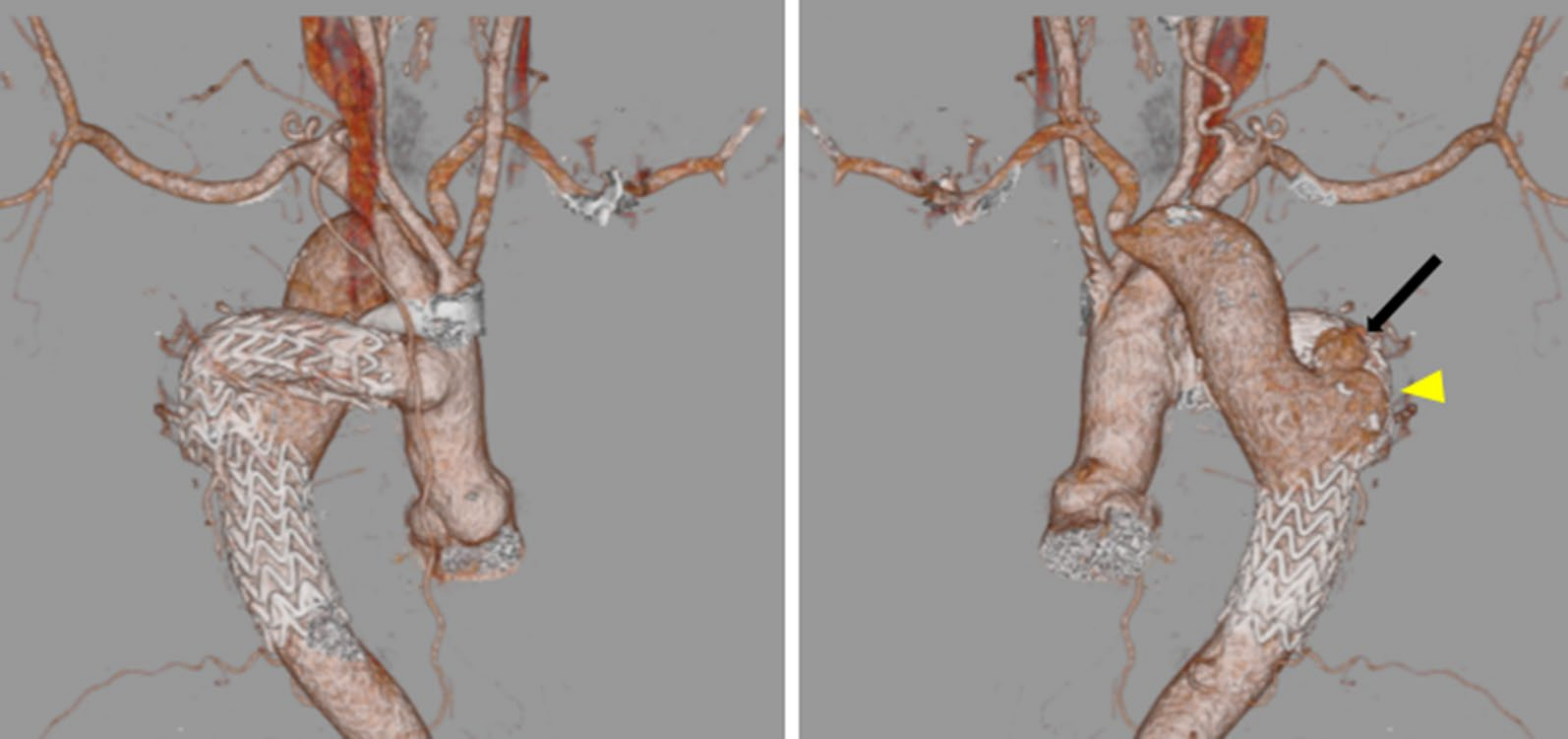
Preoperative computed tomography three-dimensional (3D) reconstruction. Anterior (a) and posterior (b) views show the right aortic arch and distal anastomotic aneurysm and contrast medium extravasation outside the aneurysm (black arrow). A small extension (similar to Kommerell diverticulum) was found in the posterior (b) view (yellow arrowhead)
